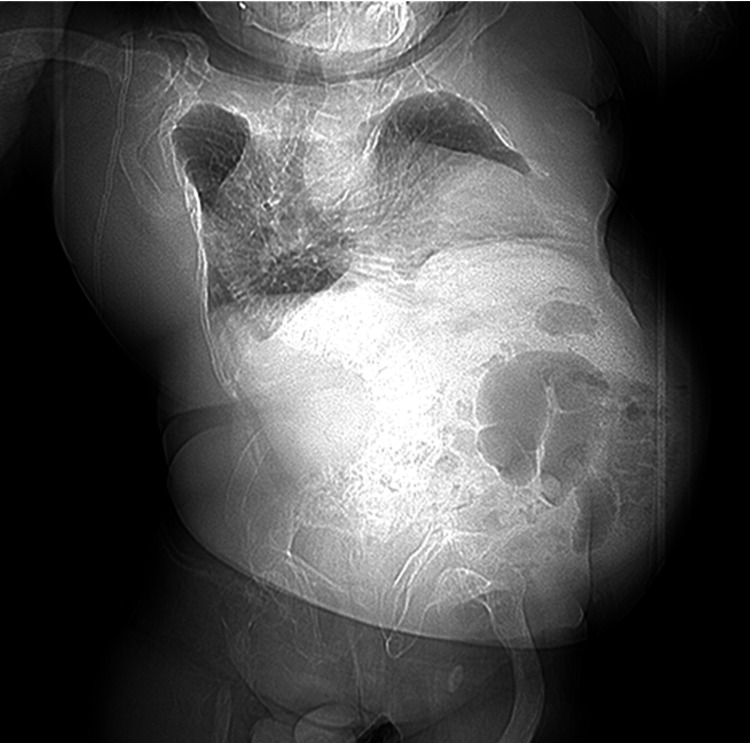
Radiography image The chest wall and spine of the patient were severely deformed.
